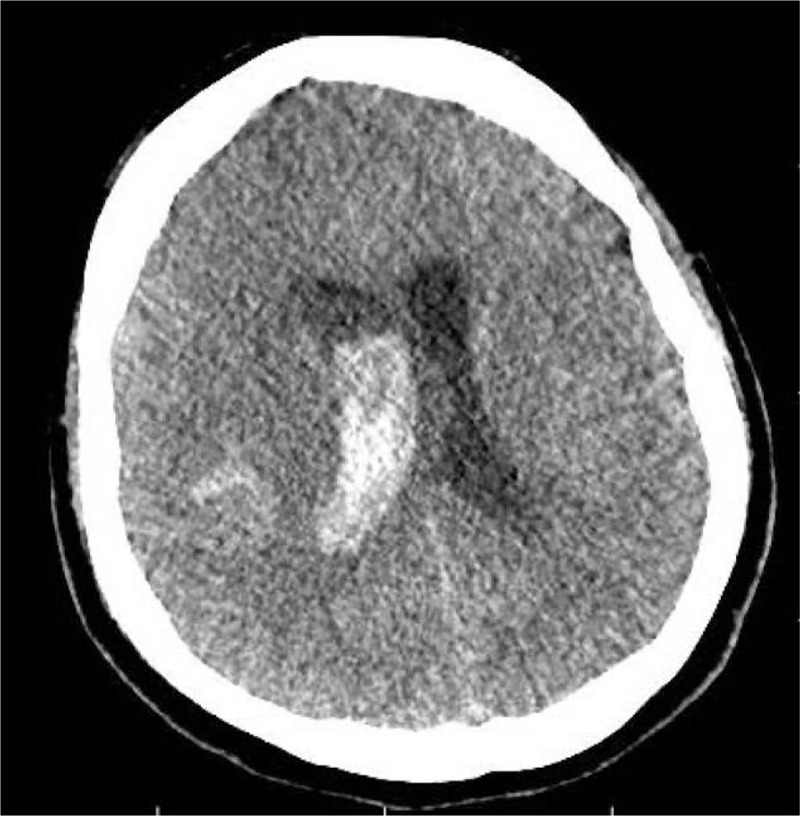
Brain CT-scan showing SAH associated with ventricular hemorrhage.
